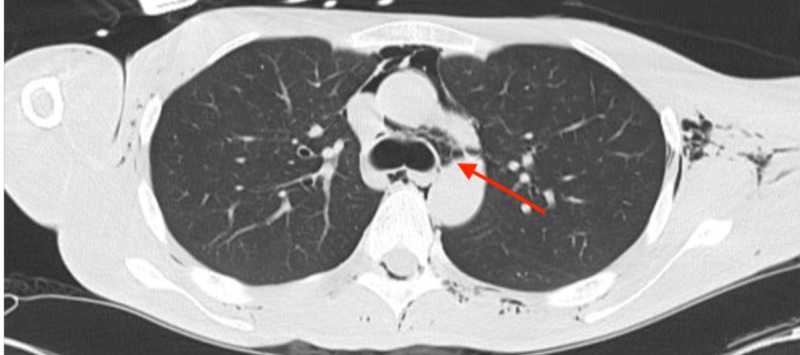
Axial slice of cervical CT highlighting pneumomediastinum, pneumorrhachis and further subcutaneous emphysema.
